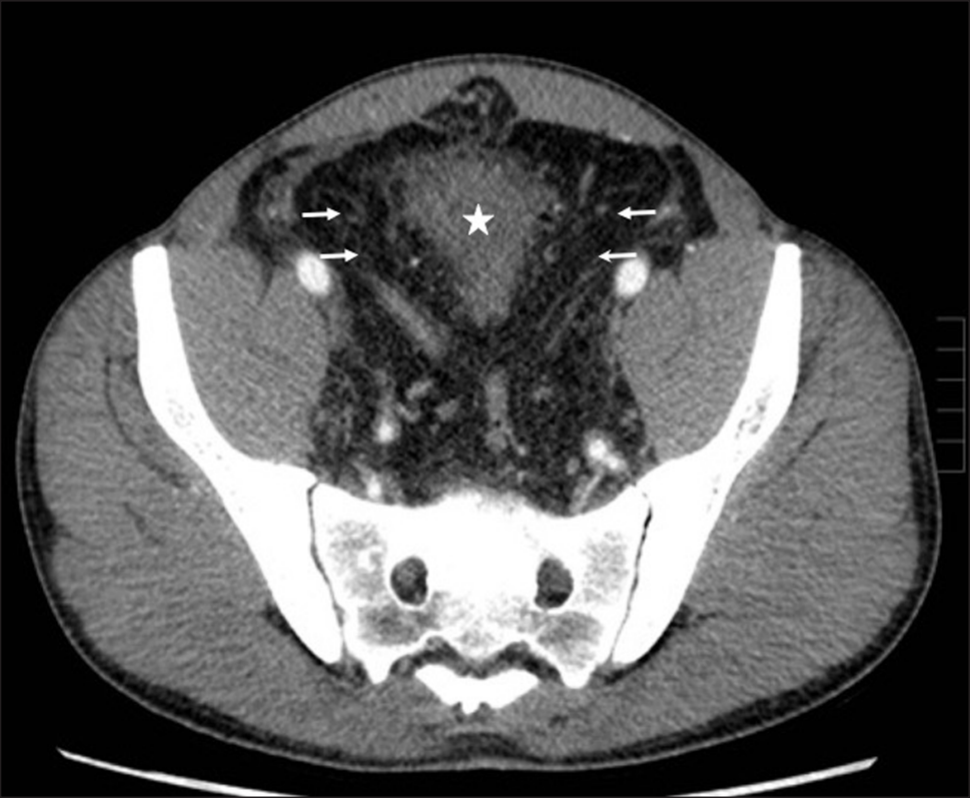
Contrast enhanced computed tomography. Axial image showing the presence of uncapsuled hypodense fat in the pelvis (arrows), causing extrinsic pressure of the bladder (white star) and the rectum.
